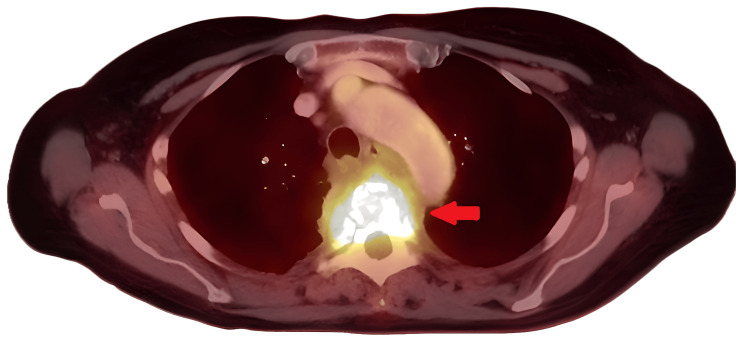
PET CT with a diagnostic CT of the neck, chest, abdomen, and pelvis with IV contrast; FDG Axial positron emission tomography/computed tomography (PET/CT) image demonstrating intense 18F-fluorodeoxyglucose (FDG) uptake (noted by the red arrow) centered at the T4–T5 disc space, with associated destruction of the adjacent vertebral bodies and extension into the posterior mediastinum and spinal canal. These findings correspond to a metabolically active, infiltrative lesion consistent with spinal osteomyelitis with imaging features suggestive of an infectious or granulomatous process.
